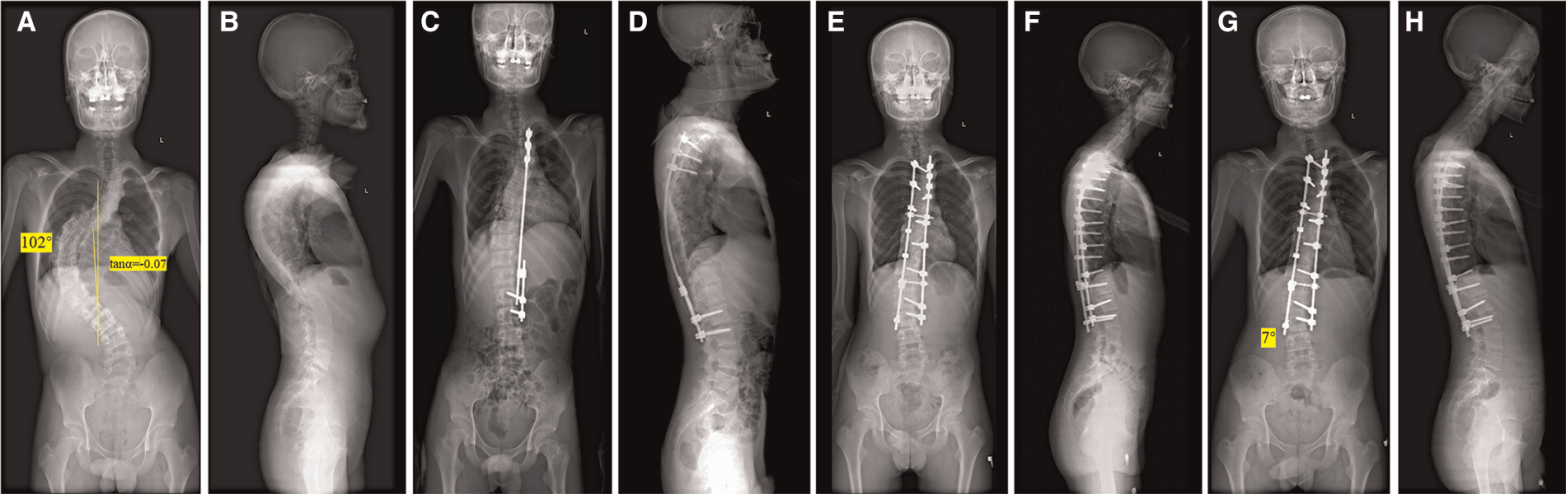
A 15-year-old boy with a severe and rigid thoracic curve (A,B). The radiographs demonstrate a thoracic curve angle of 102°, and the slope of the line connecting the pedicles on the concave side of the upper- and lower-end vertebrae (Tan *α*) is −0.07. After-distraction posteroanterior (C) and lateral (D) radiographs demonstrate well-maintained global coronal and sagittal balance. Postoperative posteroanterior (E) and lateral (F) radiographs following posterior spinal fusion from T2 to L3 demonstrate well-maintained global coronal and sagittal balance. Radiographs made at the final follow-up (G,H) reveal the appearance of the distal adding-on phenomenon with an intervertebral space angle below the LIV of 7°.
